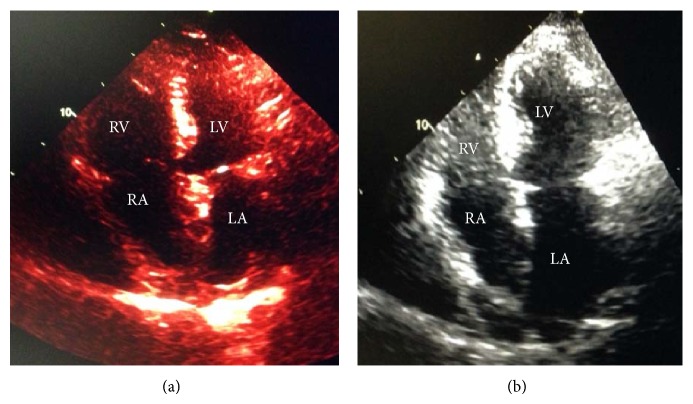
Two-dimensional transthoracic echocardiography in the apical four-chamber view. Panel (a) was obtained prior to surgical correction and demonstrates significant right atrial and ventricular enlargement. Panel (b) was obtained six months following surgical correction and demonstrates normalization of the right atrial and ventricular sizes. RA: right atrium; RV: right ventricle; LA: left atrium; LV: left ventricle.
